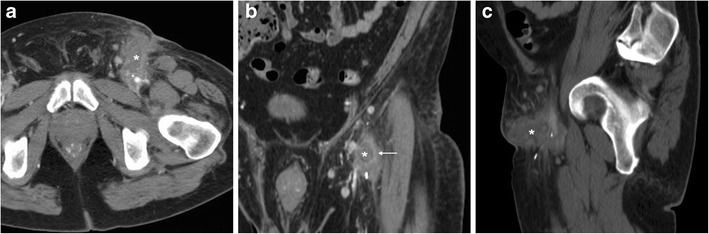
Groin abscess developing from superinfection of an iatrogenic haematoma in a 56-year-old man with acute coronary syndrome treated with PCI. Axial (A) and coronal (B) contrast-enhanced CT images showed hypoattenuating collection (*) with peripheral enhancement, extending ventrally from the CFA course. Antibiotics ultimately relieved the abscess
